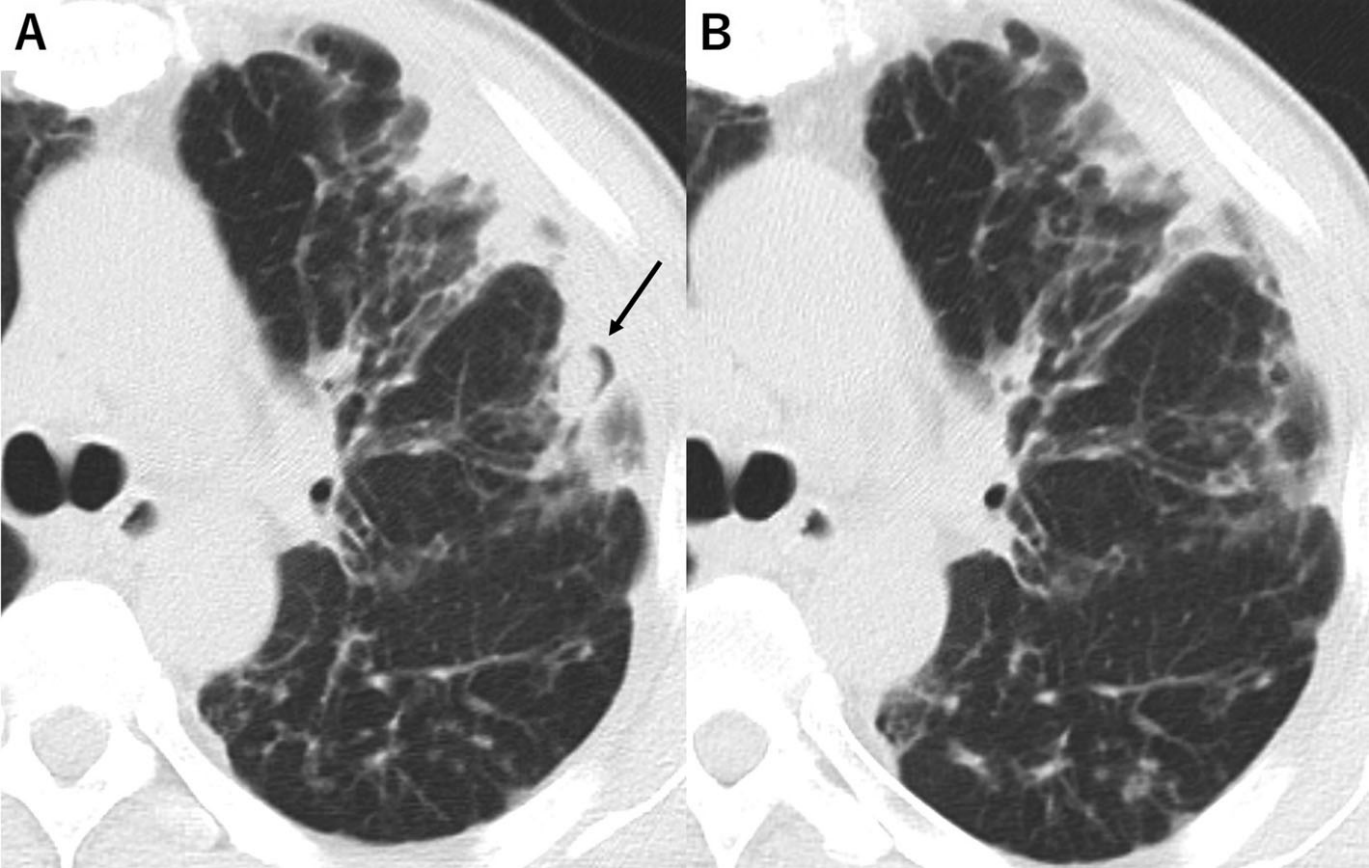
Computed tomography findings of the chest. (A) Cavitary lesions with fungus balls (arrow) were detected in the left upper lobe of the lung on admission. (B) Treatment with voriconazole for six months ameliorated the cavitary lesions.
